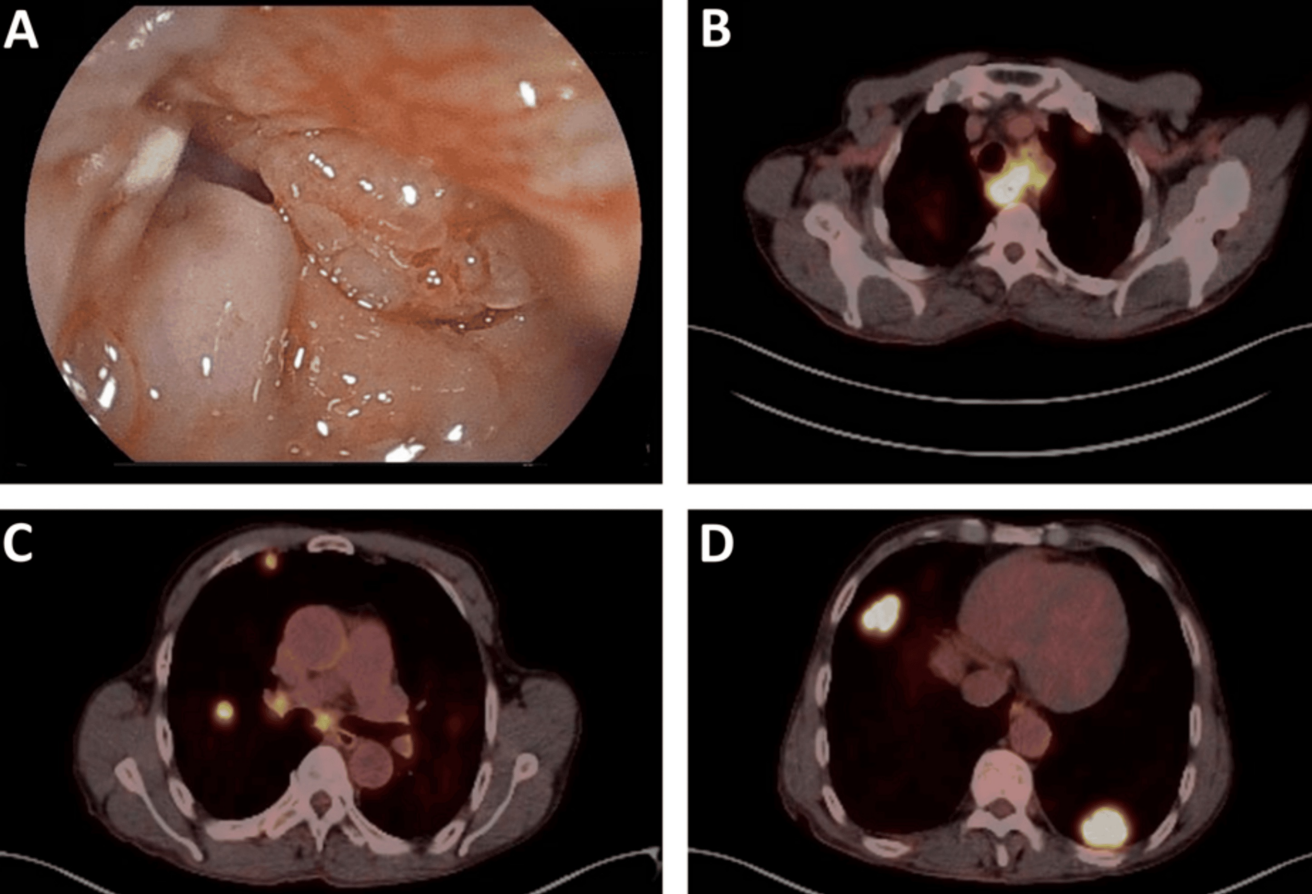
Diagnostic endoscopy and staging PET-CT imaging of dMMR proximal esophageal squamous cell carcinoma (A) Esophagogastroduodenoscopy image obtained at diagnosis in June 2023, showing a circumferential, stenosing, and rigid mass located immediately below the cricopharyngeal muscle, at 20 cm from the dental arch, consistent with esophageal carcinoma. (B) Positron emission tomography-computed tomography (PET-CT) scan (axial view) for staging, demonstrating hypermetabolic esophageal thickening at the level of T2-T4 (SUVmax 12.7), extending to the left upper paratracheal space with soft tissue density, and focal hypermetabolism in a portion of the left internal jugular vein (SUVmax 8.1). (C) PET-CT scan (coronal view) for staging, revealing bilateral mediastinal hypermetabolic lymphadenopathies (SUVmax ranging from 6.0 to 8.0) and several hypermetabolic pulmonary nodules. (D) PET-CT scan (axial view) for staging, showing dominant hypermetabolic metastases, including a 30-mm lesion in the middle lobe of the right lung (SUVmax 10.3) and a 33-mm lesion in the posterior basal segment of the left lung (SUVmax 11.9). dMMR: Mismatch repair deficiency
